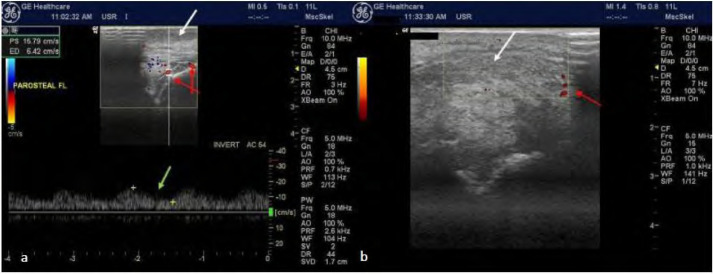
(A) CDUS of the right ankle (sagittal image of medial malleolus) showing, subcutaneous tissue oedema and posterior tibialis tendon swelling and paratendon inflammation (tenosynovitis) with hyperaemia and parosteal arterial flow. (B) CDUS of the right ankle (sagittal image of medial malleolus) 3 weeks later showing presence of mild muscle hyperaemia and significant improvement of ultrasonographic findings.
